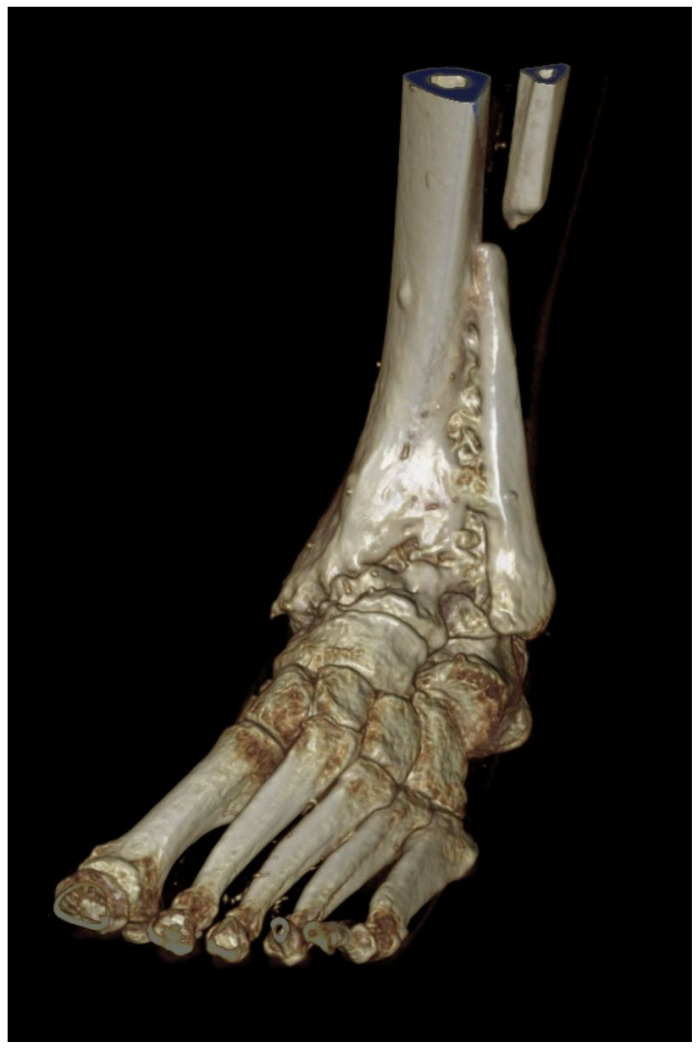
ROW-CT-3D-reconstruction 1 year after surgery. The arthrodesis of the fibula with the tibia is clearly visible.
